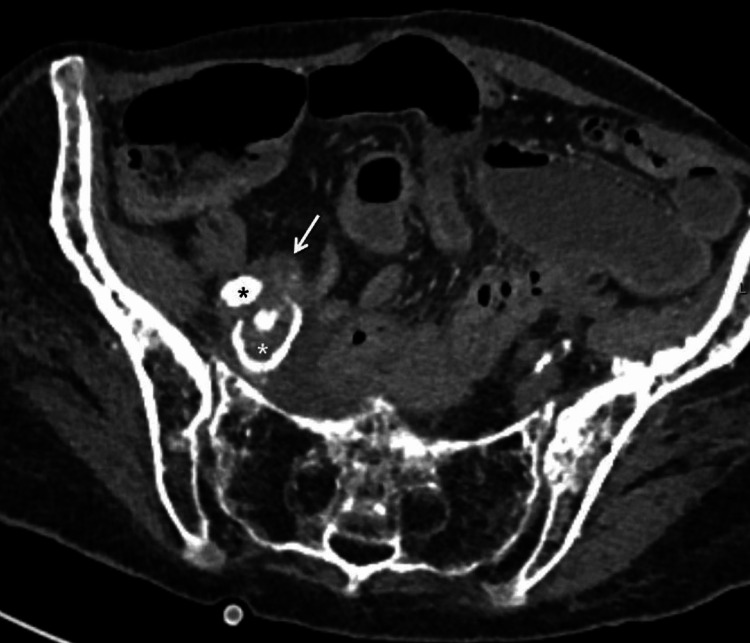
Axial CT angiogram. The ureter (white arrow) is inseparable from the external iliac artery (black asterisk) and ectatic internal iliac artery (white asterisk). No active extravasation of contrast seen at the time of the scan.
